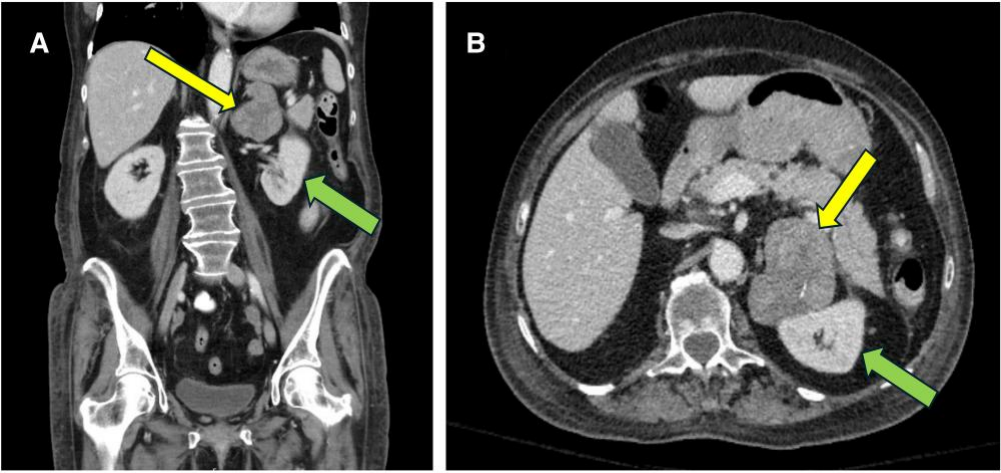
Preoperative CT of the abdomen with and without contrast in coronal view (A) and axial view (B) showing a 7.1 × 5.8 × 5.9-cm heterogenous left adrenal mass (yellow arrow). The mass was mildly heterogenous, contained a few internal calcifications, and had proximity to the left kidney (green arrow). The mass had an unenhanced attenuation of 25 Hounsfield units, 54 Hounsfield units during the venous phase, and 40 Hounsfield units postcontrast. The washout characteristics were indeterminate.
